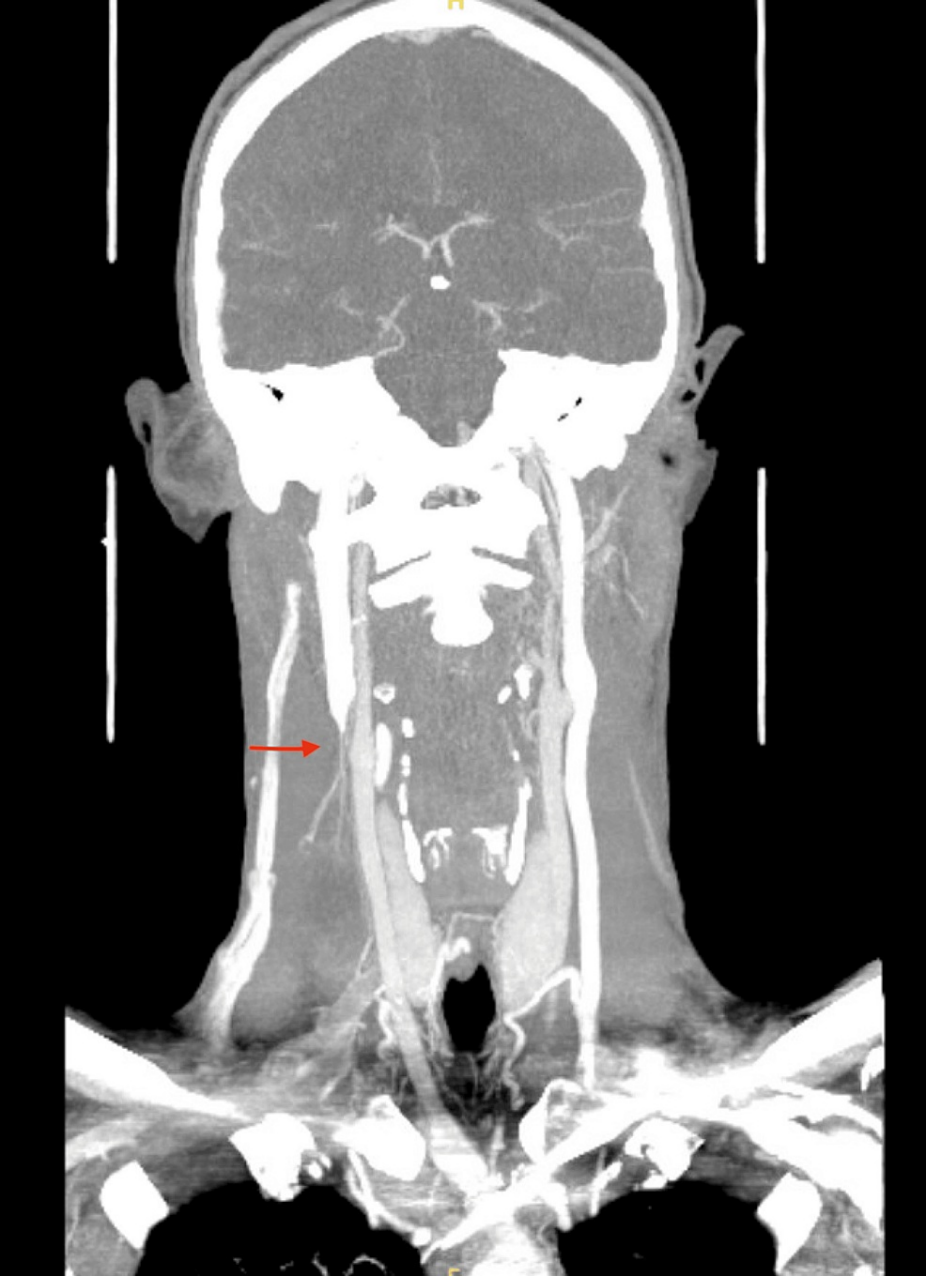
CT venogram of the head and neck The red arrow depicts an occlusive thrombus of the right internal jugular vein, which extends from the level of C3 inferiorly to the right brachiocephalic vein, superior vena cava above the level of the azygos vein, and occluded right subclavian vein.
